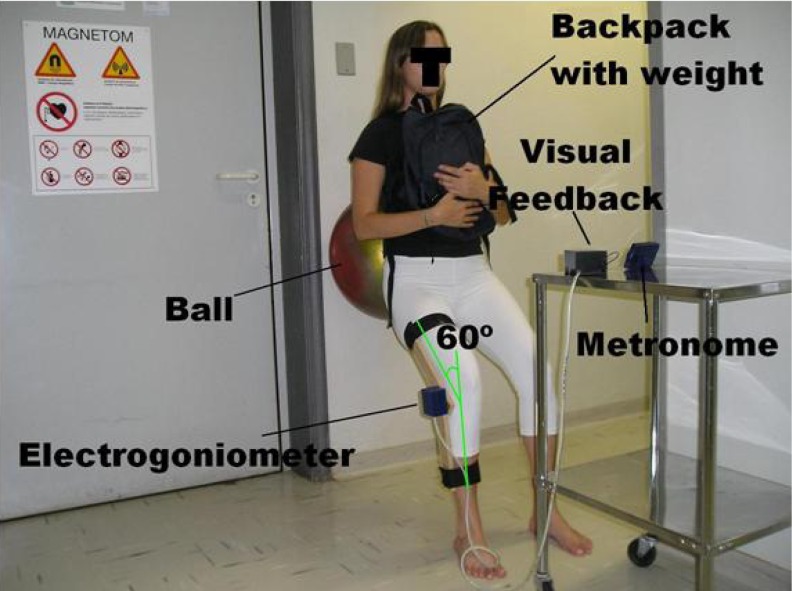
Execution of the squat exercise supported by a ball between the lumbar of the volunteer and the wall of the MRI shielded room. The metronome and light (LED) of the electrogoniometer, which indicates when the maximum flexion was attained, were placed on a table in front of the volunteer
